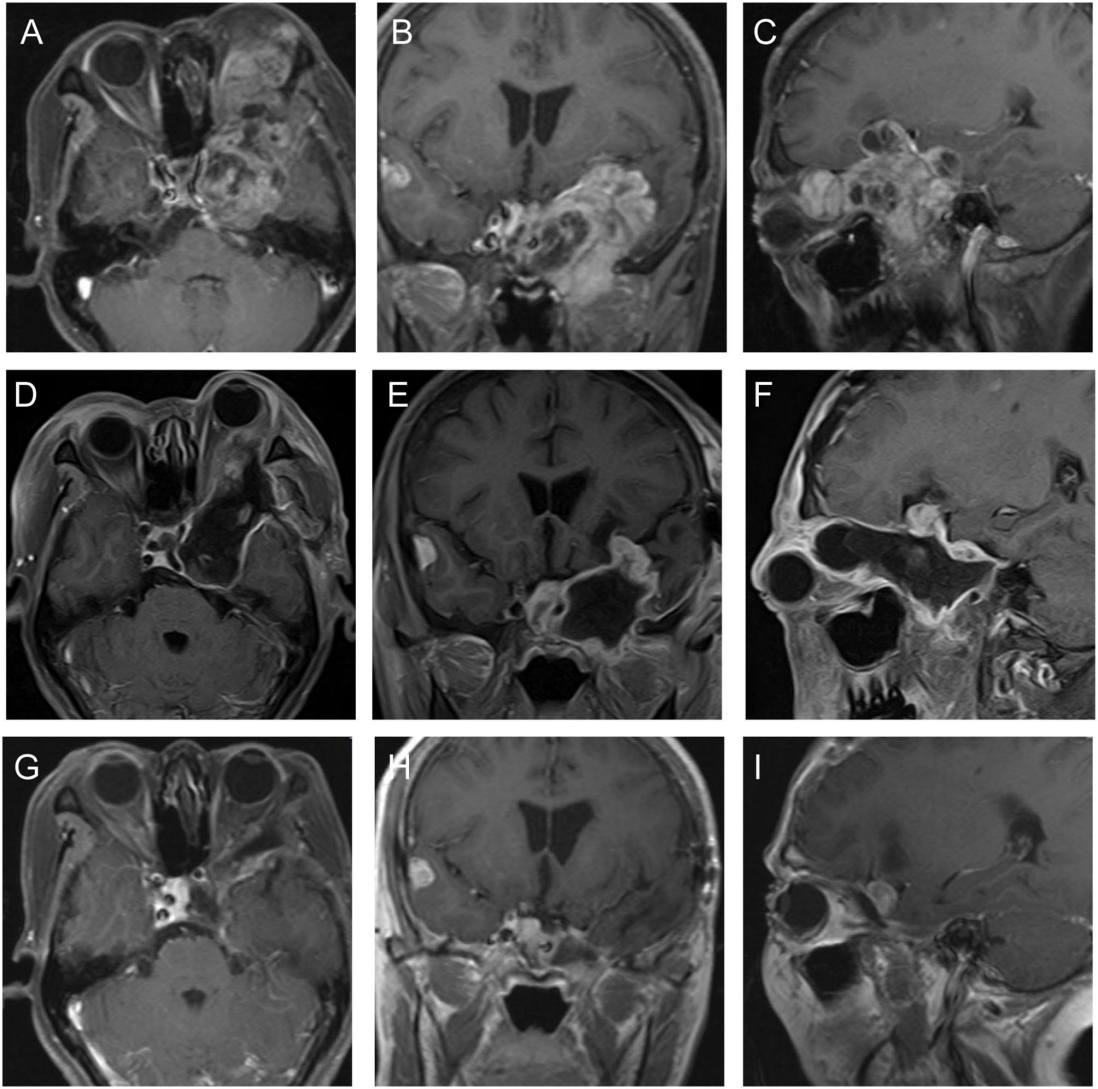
The preoperative, postoperative, and follow-up MRI imaging of case 5. (A–C) Preoperative MRI showed the trigeminal schwannoma involved the left middle fossa, orbit, and infratemporal fossa. (D–F) Postoperative MRI showed the most of the tumor was completely removed. We considered the postoperative dura enhancement was due to the scarring formation from the primary surgery. (G–I) Follow-up MRI showed no recurrence.
